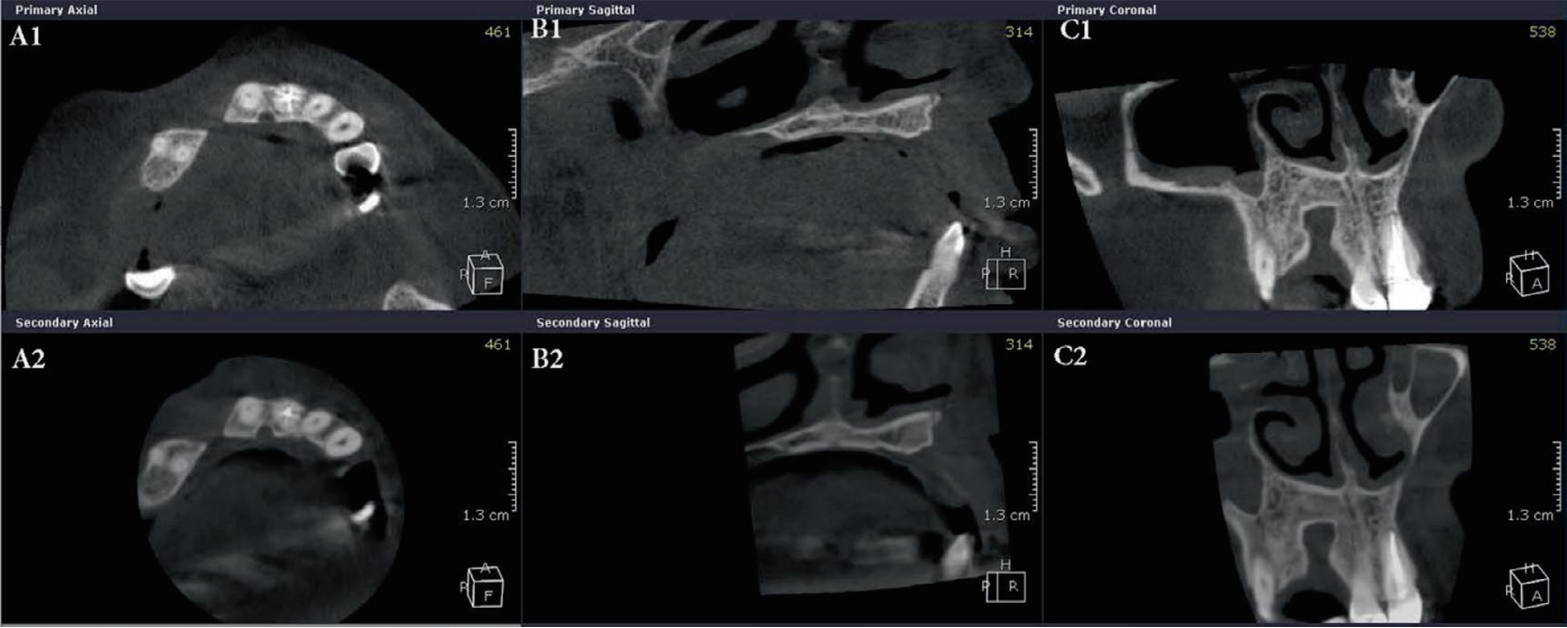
CBCT images show a well‐defined and corticated bony defect in the maxilla between Teeth 11 and 14. Taken from (A) axial, (B) sagittal, and (C) coronal sections. First row (A1), (B1), (C1) are images taken at the 5‐year follow‐up postsurgical resection. Second row images (A2), (B2), (C2) are taken at the 10‐year follow‐up postresection, indicating the stability of the lesion.
